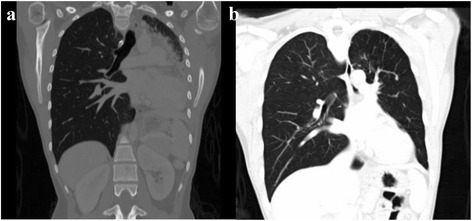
CT Thorax pre- and post-intervention. a Coronal view CT Thorax showed total left lung collapse. b Coronal view CT Thorax at 36 weeks following intervention showing re-expansion of the left lung
